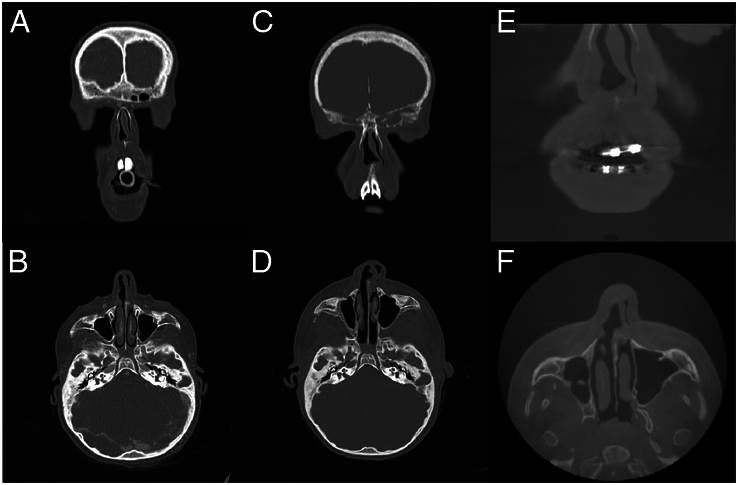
A-F Example of a patient with worsened nasal asymmetry postoperatively. Patient underwent MB with internal distractors at 10 years old. Clinically, preoperatively a clinically deviated nose was observed, on the short-term postoperative facial photograph a worsened deviation was observed, and on the long-term facial photograph an improvement of the nasal deviation was observed. The clinical findings were align with the findings on the CT scans: A. preoperative frontal view showing a small caudal septal dislocation at the level of the ANS, B. preoperative axial view showing the preoperative position, C. 4 month postoperative frontal view showing an increased caudal septal dislocation, D. 4 months postoperative axial view showing a dislocation of the cartilaginous septum and flattening of the external nose, E. 4 years postoperative frontal view showing a slightly reduced caudal septal dislocation without surgery, F. 4 years postoperative axial view still showing dislocation of the cartilaginous septum but improved position of the external nose.
